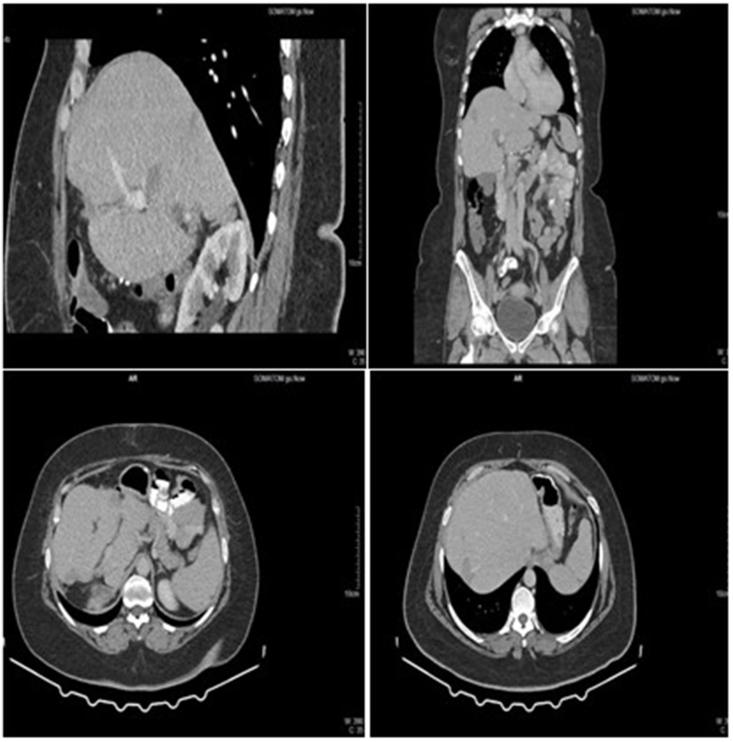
A computed tomography scan after 30 months of initiation of pazopanib revealed a response in a patient with primary hepatic epithelioid hemangioendothelioma. The scan revealed a triangular hypodense lesion in the periphery of the seventh hepatic segment which demonstrated a partial response. In addition, there is a right adrenal mass measuring 2.5 cm, which is consistent with a benign adenoma (stable compared with previous scans).
